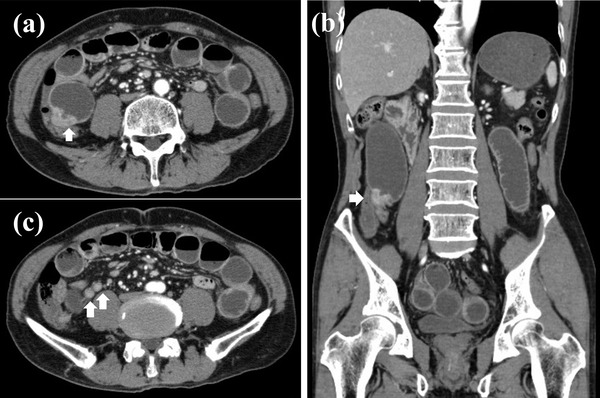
Abdominal computed tomography findings. (a,b) The tumor caused intestinal obstruction (c) accompanied by enlarged lymph nodes. White arrows in (a) and (b) indicate the main lesion, and those in (c) indicate the swollen lymph nodes
